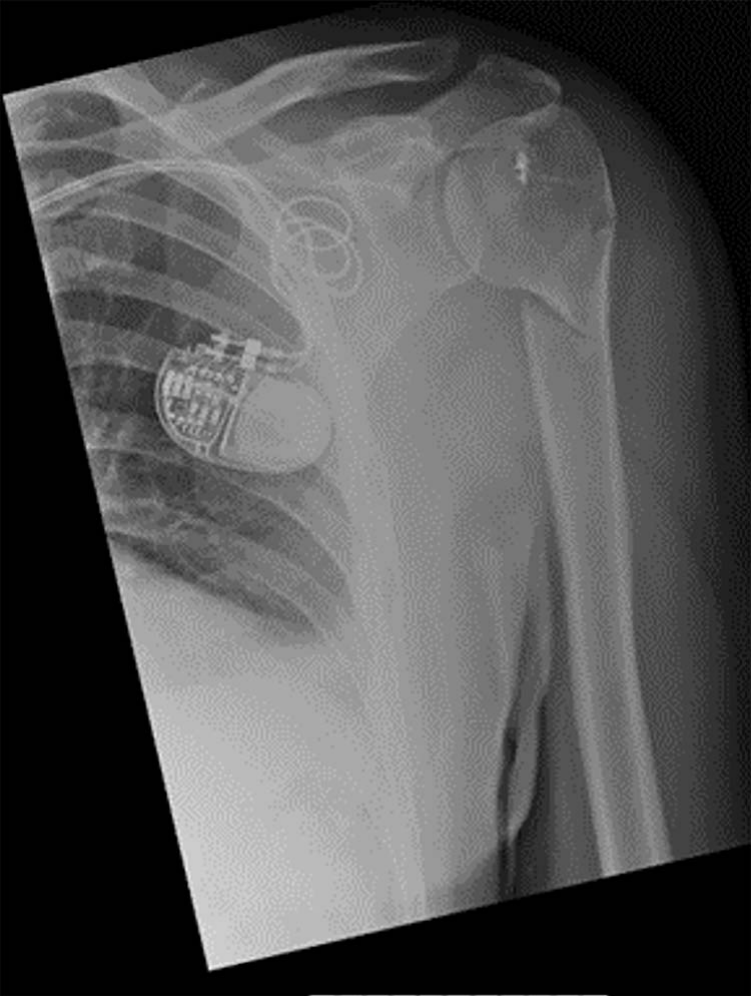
Antero-posterior view X-ray taken 1 week post-injury showing acceptable positioning of the fracture.
